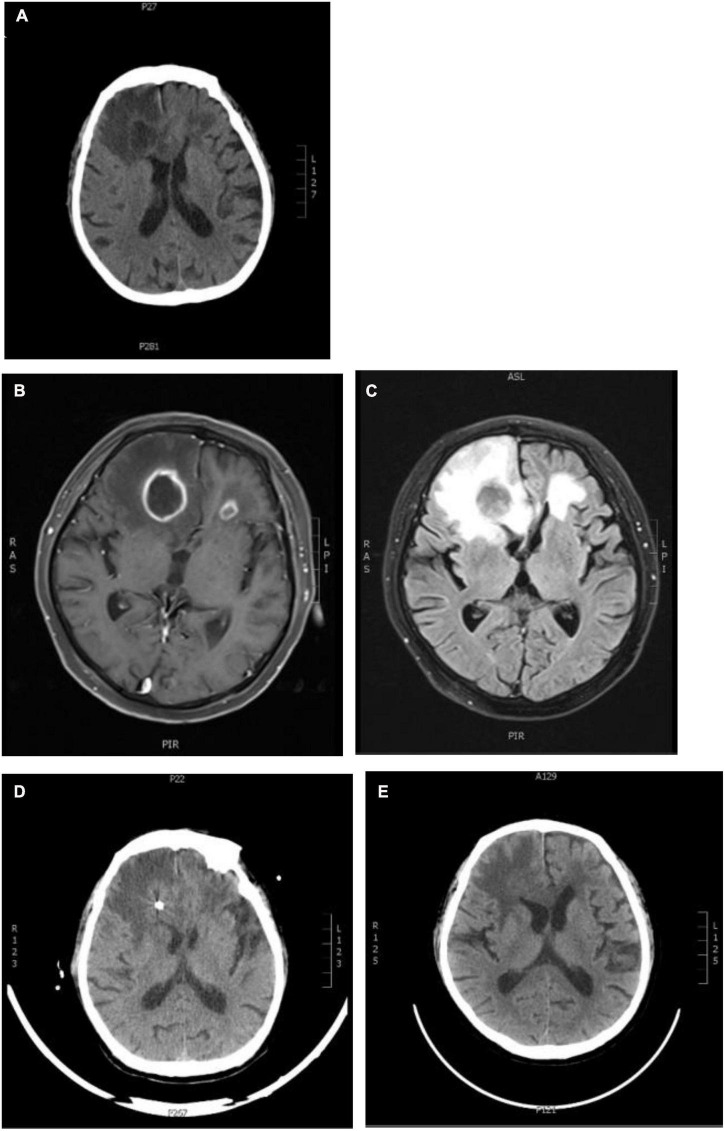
Changes in brain imaging. (A) Craniocerebral CT: The patchy low-density shadows were seen in the bilateral frontal and temporal lobes (the right side was the indigenous), and the patchy low-density shadows were also seen in the bilateral basal ganglia and paraventricular zone. The density of white matter in the paraventricular zone was reduced, and there was no abnormal density change in the cerebellum and brainstem; (B,C) craniocerebral MRI + enhancement + diffusion: bilateral frontal lobe abscess; (D,E) craniocerebral CT after abscess puncture and drainage: postoperative changes, bilateral frontal lobe multiple enhancement lesions, and some lesions shrink.
